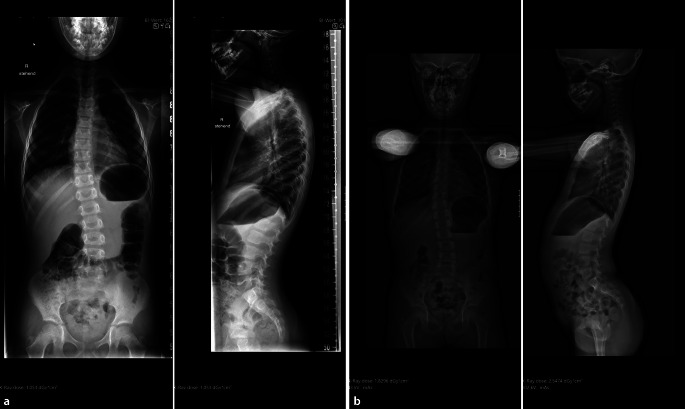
In den letzten Jahrzehnten haben viele technische Innovationen der Röntgen‑, Durchleuchtungs- und CT-Geräte zu einer erheblichen Strahlenreduktion im gesamten Bereich der Kinderradiologie geführt. Zudem konnte dank des Ultraschalls und der MRT das Strahlenrisiko bei Kindern so weit reduziert werden, dass kein Schaden durch die Bildgebung für den Patienten zu erwarten ist. Ein DosisüberwachungssystemDosisüberwachungssystem ist empfehlenswert, um die Röntgendosis von verschiedenen Gewichts- oder Altersgruppen jeweils optimieren zu können und die Parameter und Strahlenbelastung an neue Geräte oder Innovationen (z. B. Photon-Counting-Detektoren) anpassen zu können. Um die korrekte effektive Dosis dem Patienten zuordnen zu können, ist es notwendig, für jede Röntgenuntersuchung das Gewicht und die Größe des Patienten für das Dosisüberwachungssystem zu erfassen. Diese Werte können automatisch von der Messstation an das System übermittelt und regelmäßig für verschiedene Gewichtsgruppen kontrolliert werden. Dabei spielen DosisreferenzwerteDosisreferenzwerte eine immer wichtigere Rolle, die mit dem Dosisüberwachungssystem einfach kontrolliert und eingehalten werden können.
